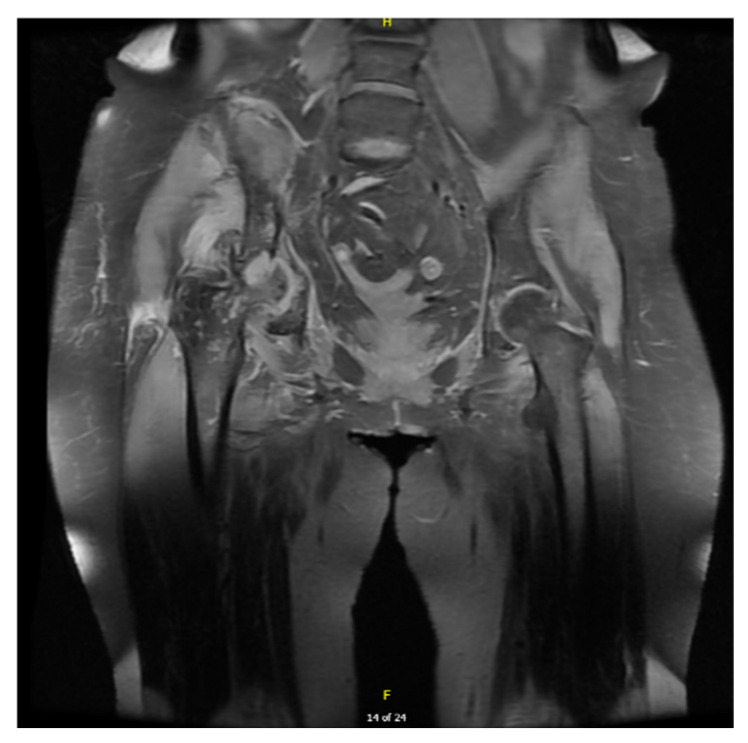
Coronal T1-weighted contrast-enhanced MRI of the pelvis demonstrating aseptic necrosis of the femoral head with surrounding bone marrow changes.
